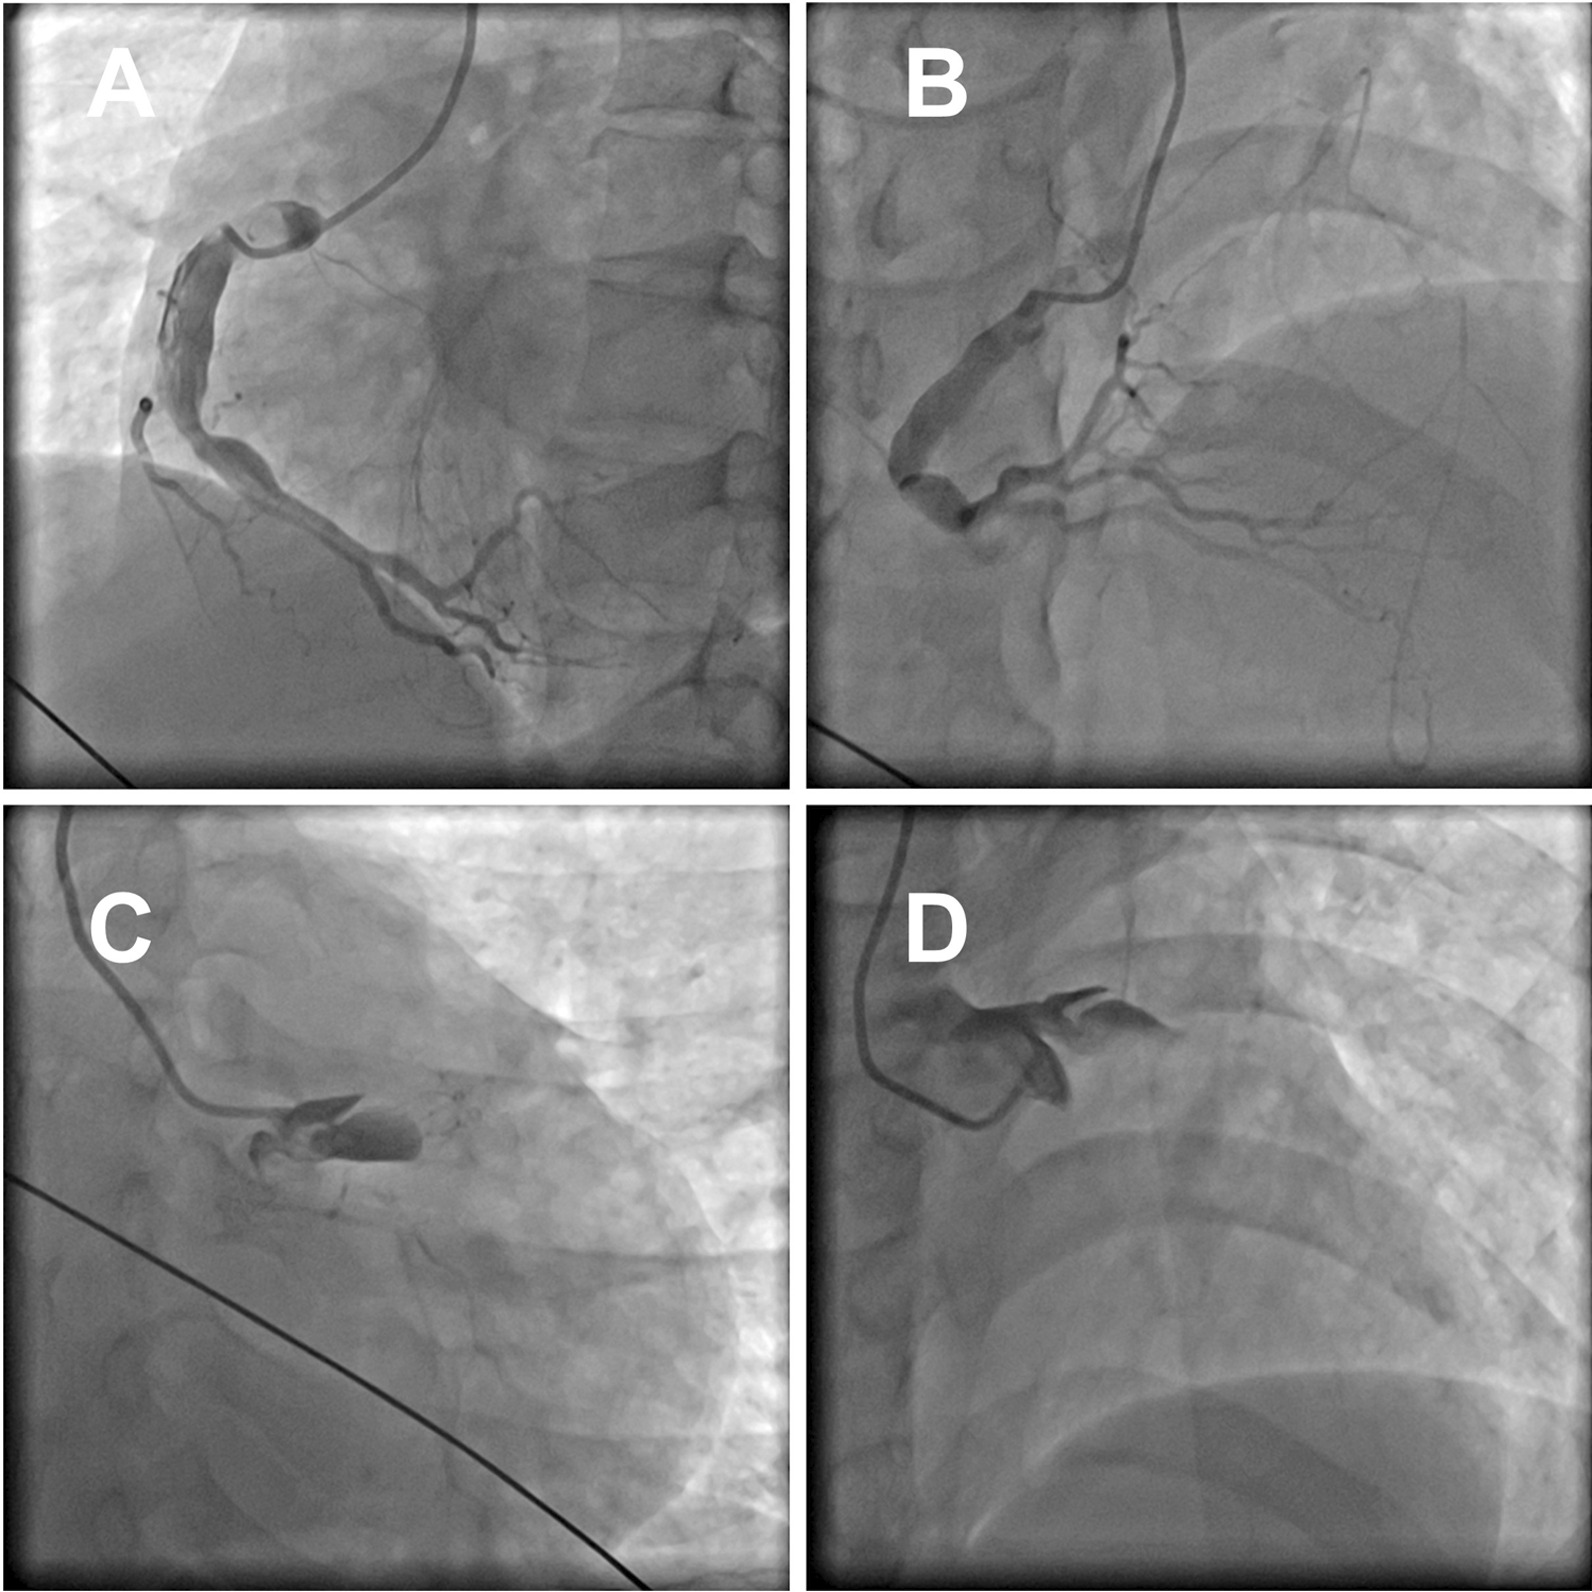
Emergency coronary CT angiography showing right coronary dilatation, spontaneous left main dissection
